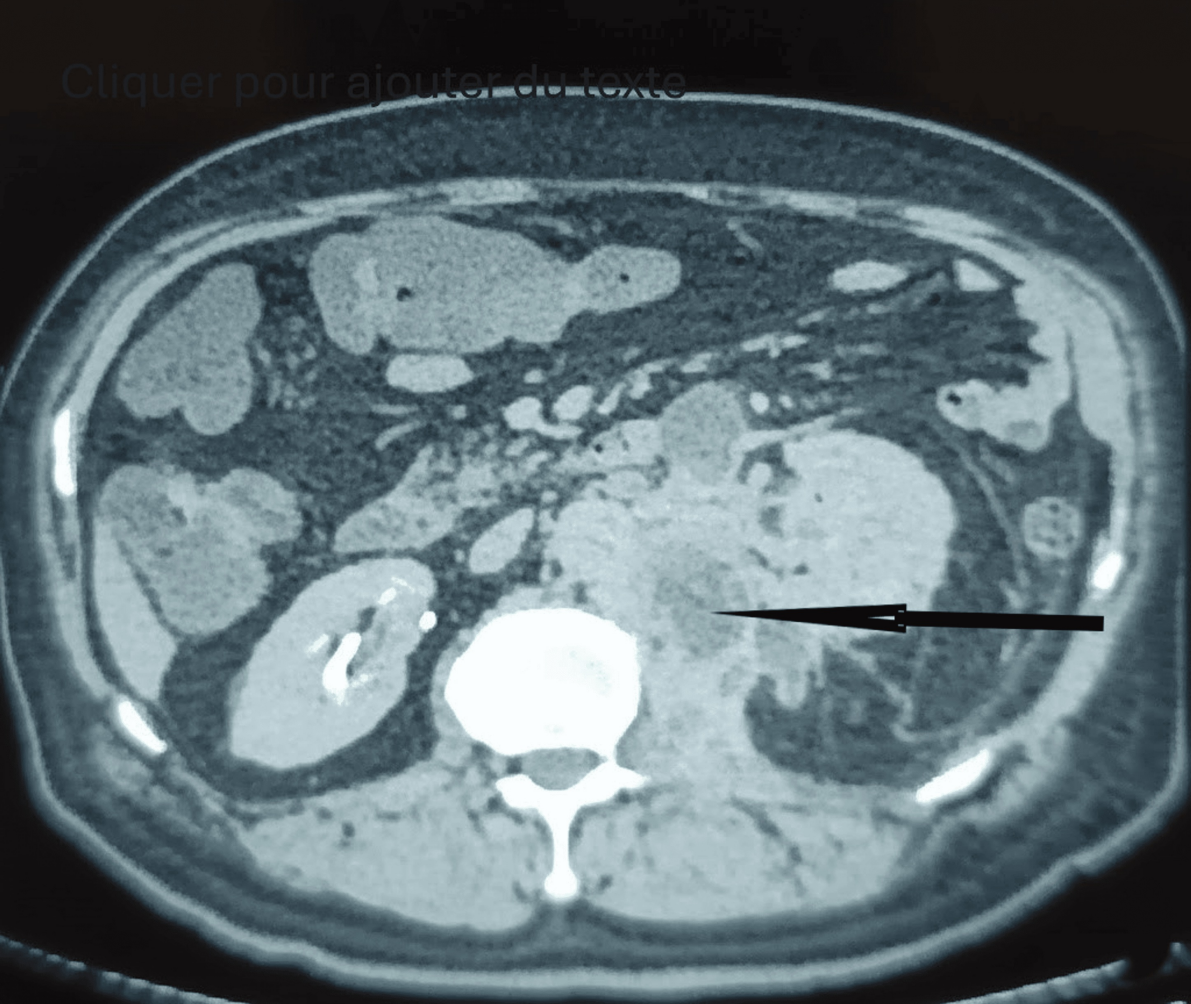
CT scan shows the infiltration of the lumbar portion of the left psoas muscle, which appears enlarged and heterogeneous, containing multiple fluid collections (arrow).
